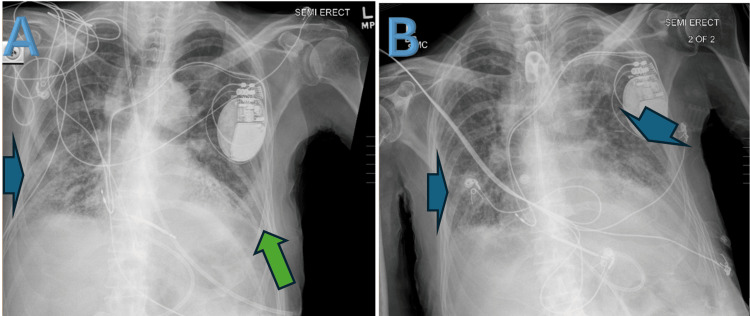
Chest X-ray Panel A showing bilateral hazy airspace opacities (blue arrow) with small pleural effusions (green arrow). Panel B showing stable mild cardiomegaly and improving pulmonary edema with increased aeration (blue arrows).
